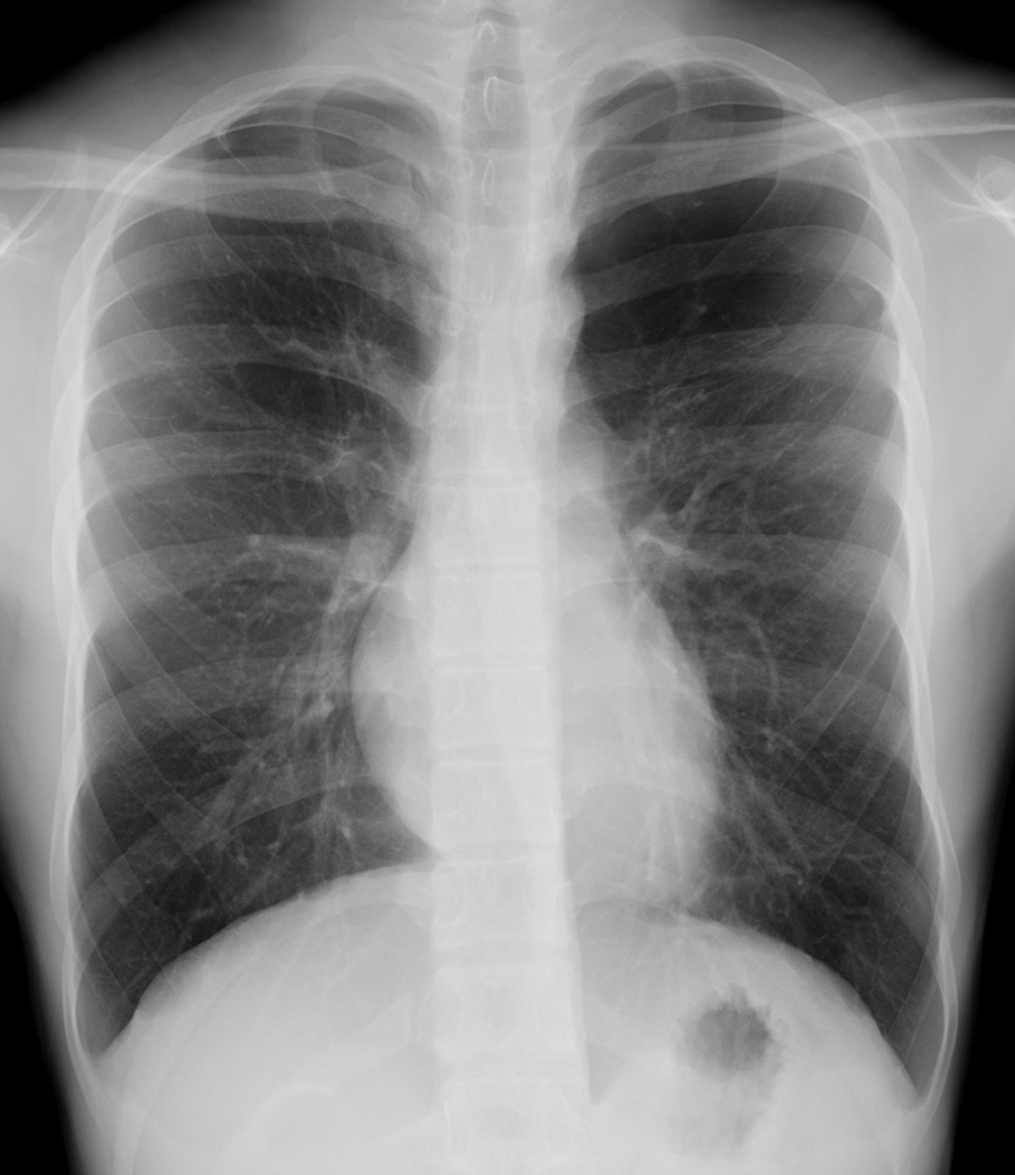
Chest radiograph showing increased radiolucency in the left upper lung field.
